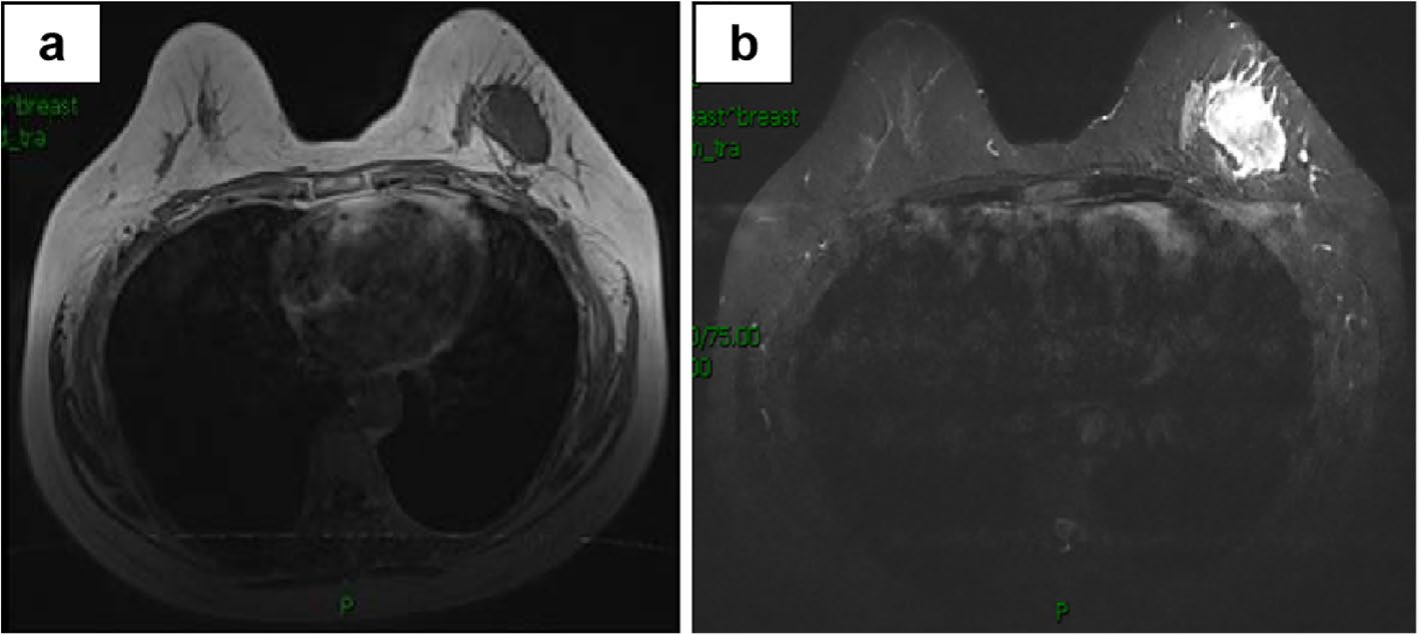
MRI revealed a mass measuring 42 × 29 × 28 mm in the upper outer quadrant of the left breast. a On T1 weighted images, the mass was isointense relative to the parenchyma. b On T2 weighted images, the mass was slightly hyperintense relative to the parenchyma
